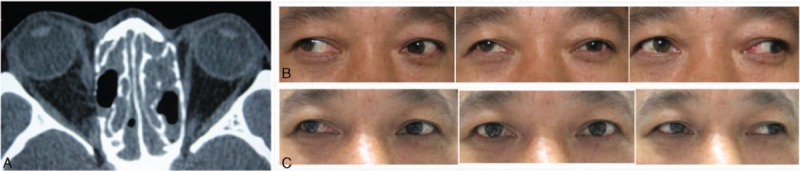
Clinical photos of case 11. (A) Axial computed tomography view showing right medial rectus transection in the posterior 3rd with small amounts of the posterior muscle segment remaining intact. (B) Preoperative clinical photos showing right exotropia of 35 prism diopters and obvious limitations of adduction. (C) Postoperative clinical photos after lateral rectus recession 8 mm and medial rectus resection 6 mm. No horizontal heterotropia was noted postoperatively in the primary position with only mild limitations of adduction.
